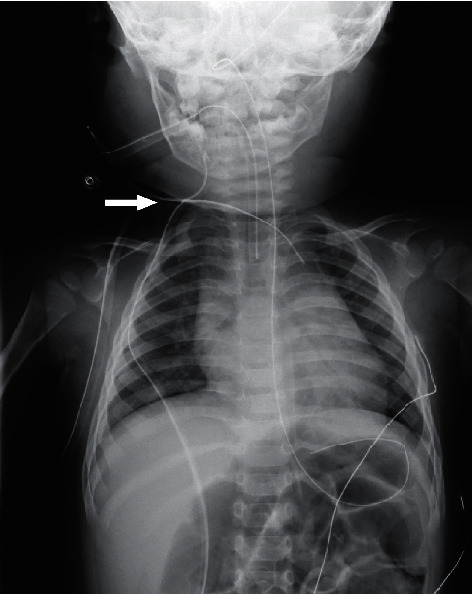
Chest radiograph after percutaneous drainage tubes were placed. The white arrow shows the insertion site. One was placed in the right neck, and the other was placed in the anterior mediastinum.
